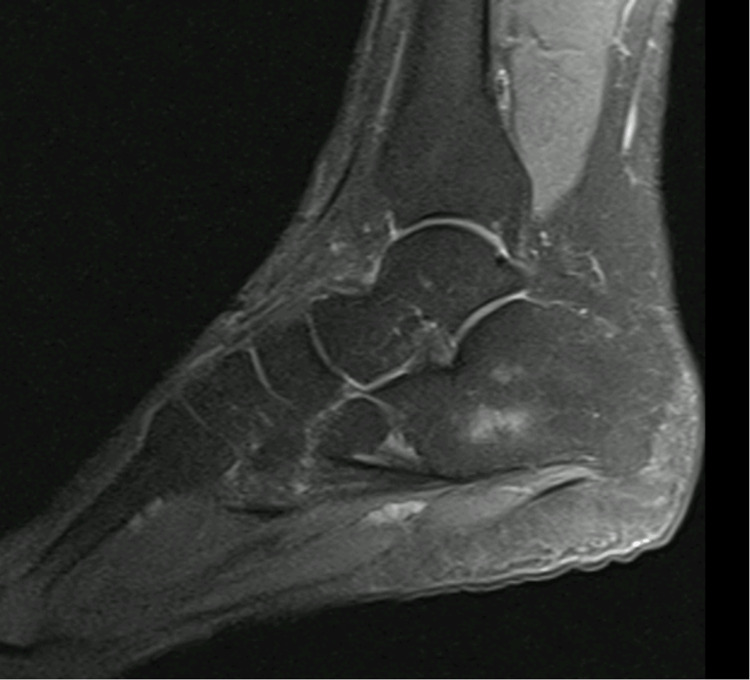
MRI image showing complete removal of the schwannoma
